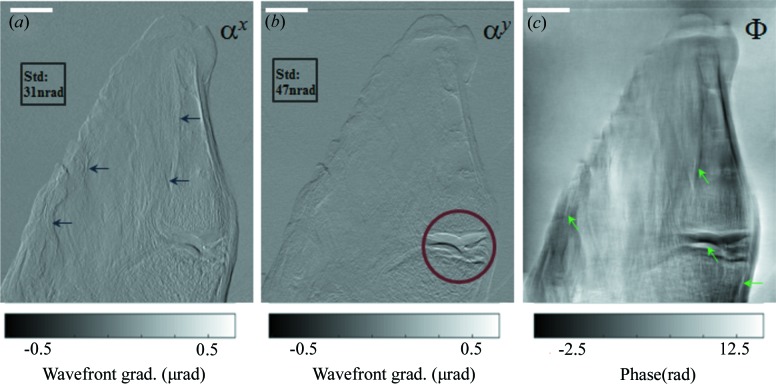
Hard X-ray speckle technique applied to the imaging of a chicken’s wing tip. (*a*) Horizontal and (*b*) vertical wavefront gradients, (*c*) phase contrast obtained with the speckle tracking technique. The scale bar at the top of image is 3.0 mm long.
